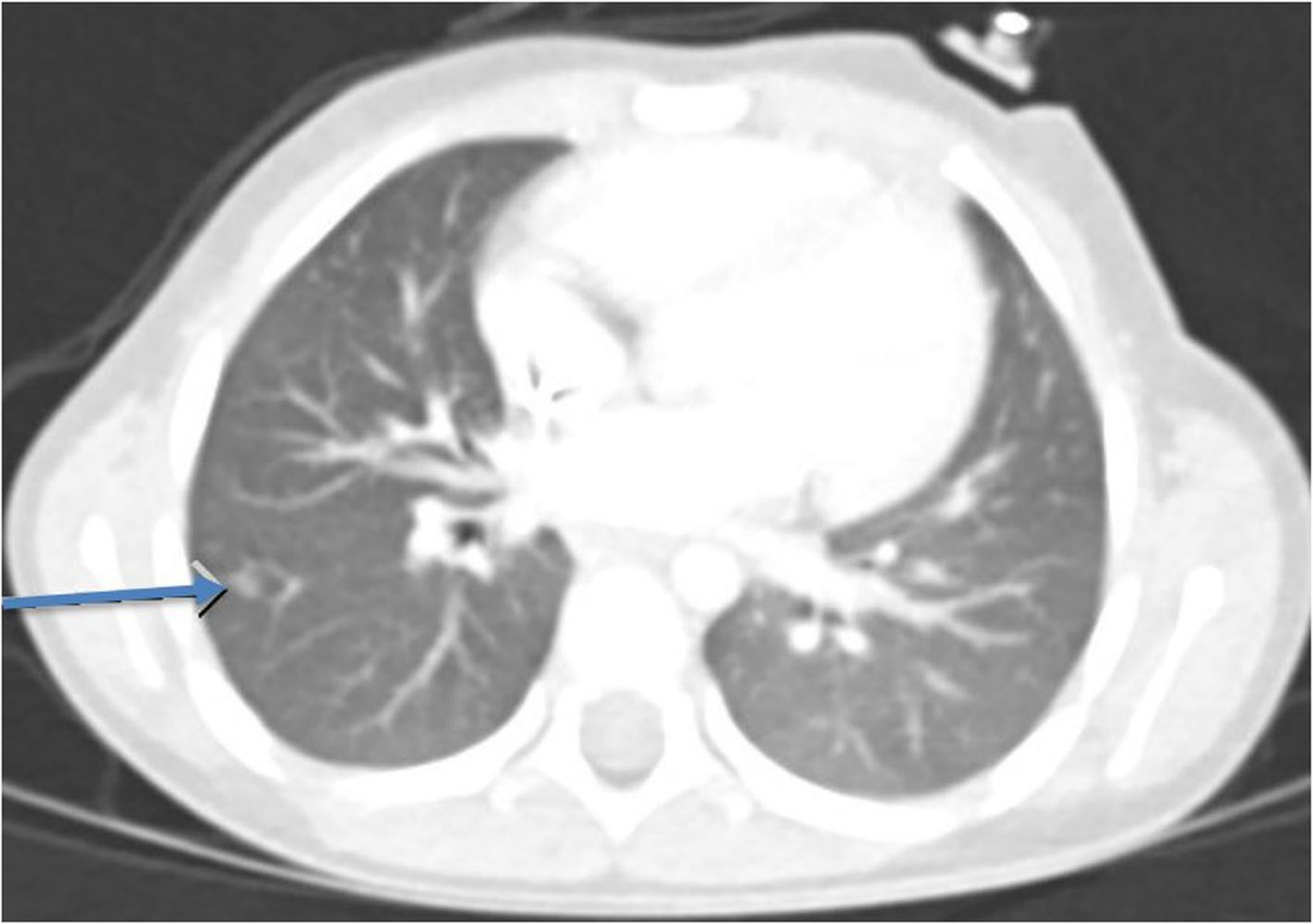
Transverse chest CT image of a 4-year-old girl (15 kg, CTDIvol 1.4 mGy). There is a subcentimeter nodule in the right lower lobe (arrow). Median IQSC score was 3
